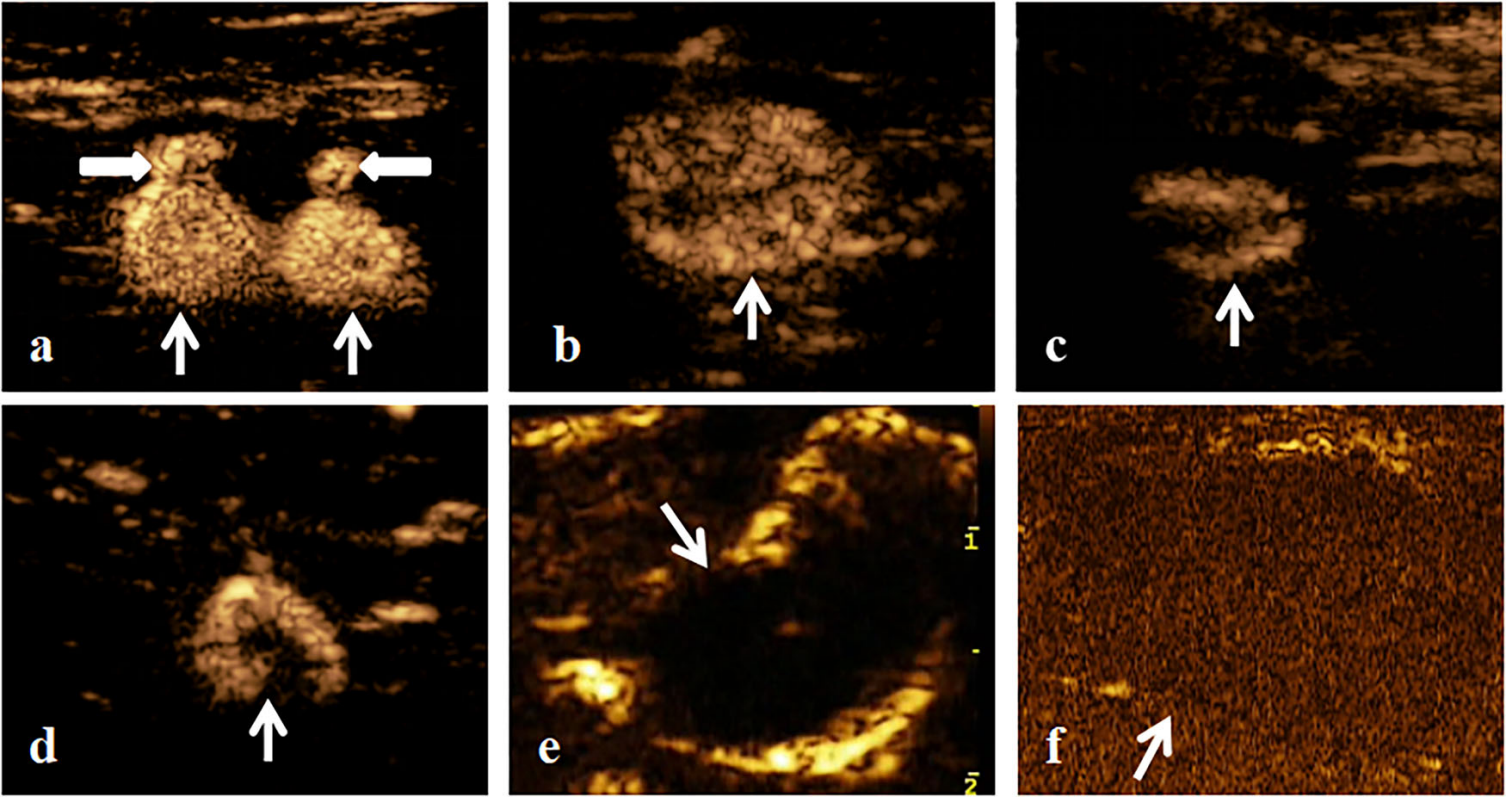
The CEUS enhancement patterns of SLN. The thin white arrows represent the SLN, the thick white arrows represent the short-axis section of LC: a Homogeneous enhancement. b–e Heterogeneous enhancement. f No enhancement. CEUS, contrast-enhanced ultrasound; SLN, sentinel lymph node; LC, lymphatic channel. Homogeneous enhancement indicates no SLN metastasis; heterogeneous or no enhancement suggests the presence of SLN metastasis
